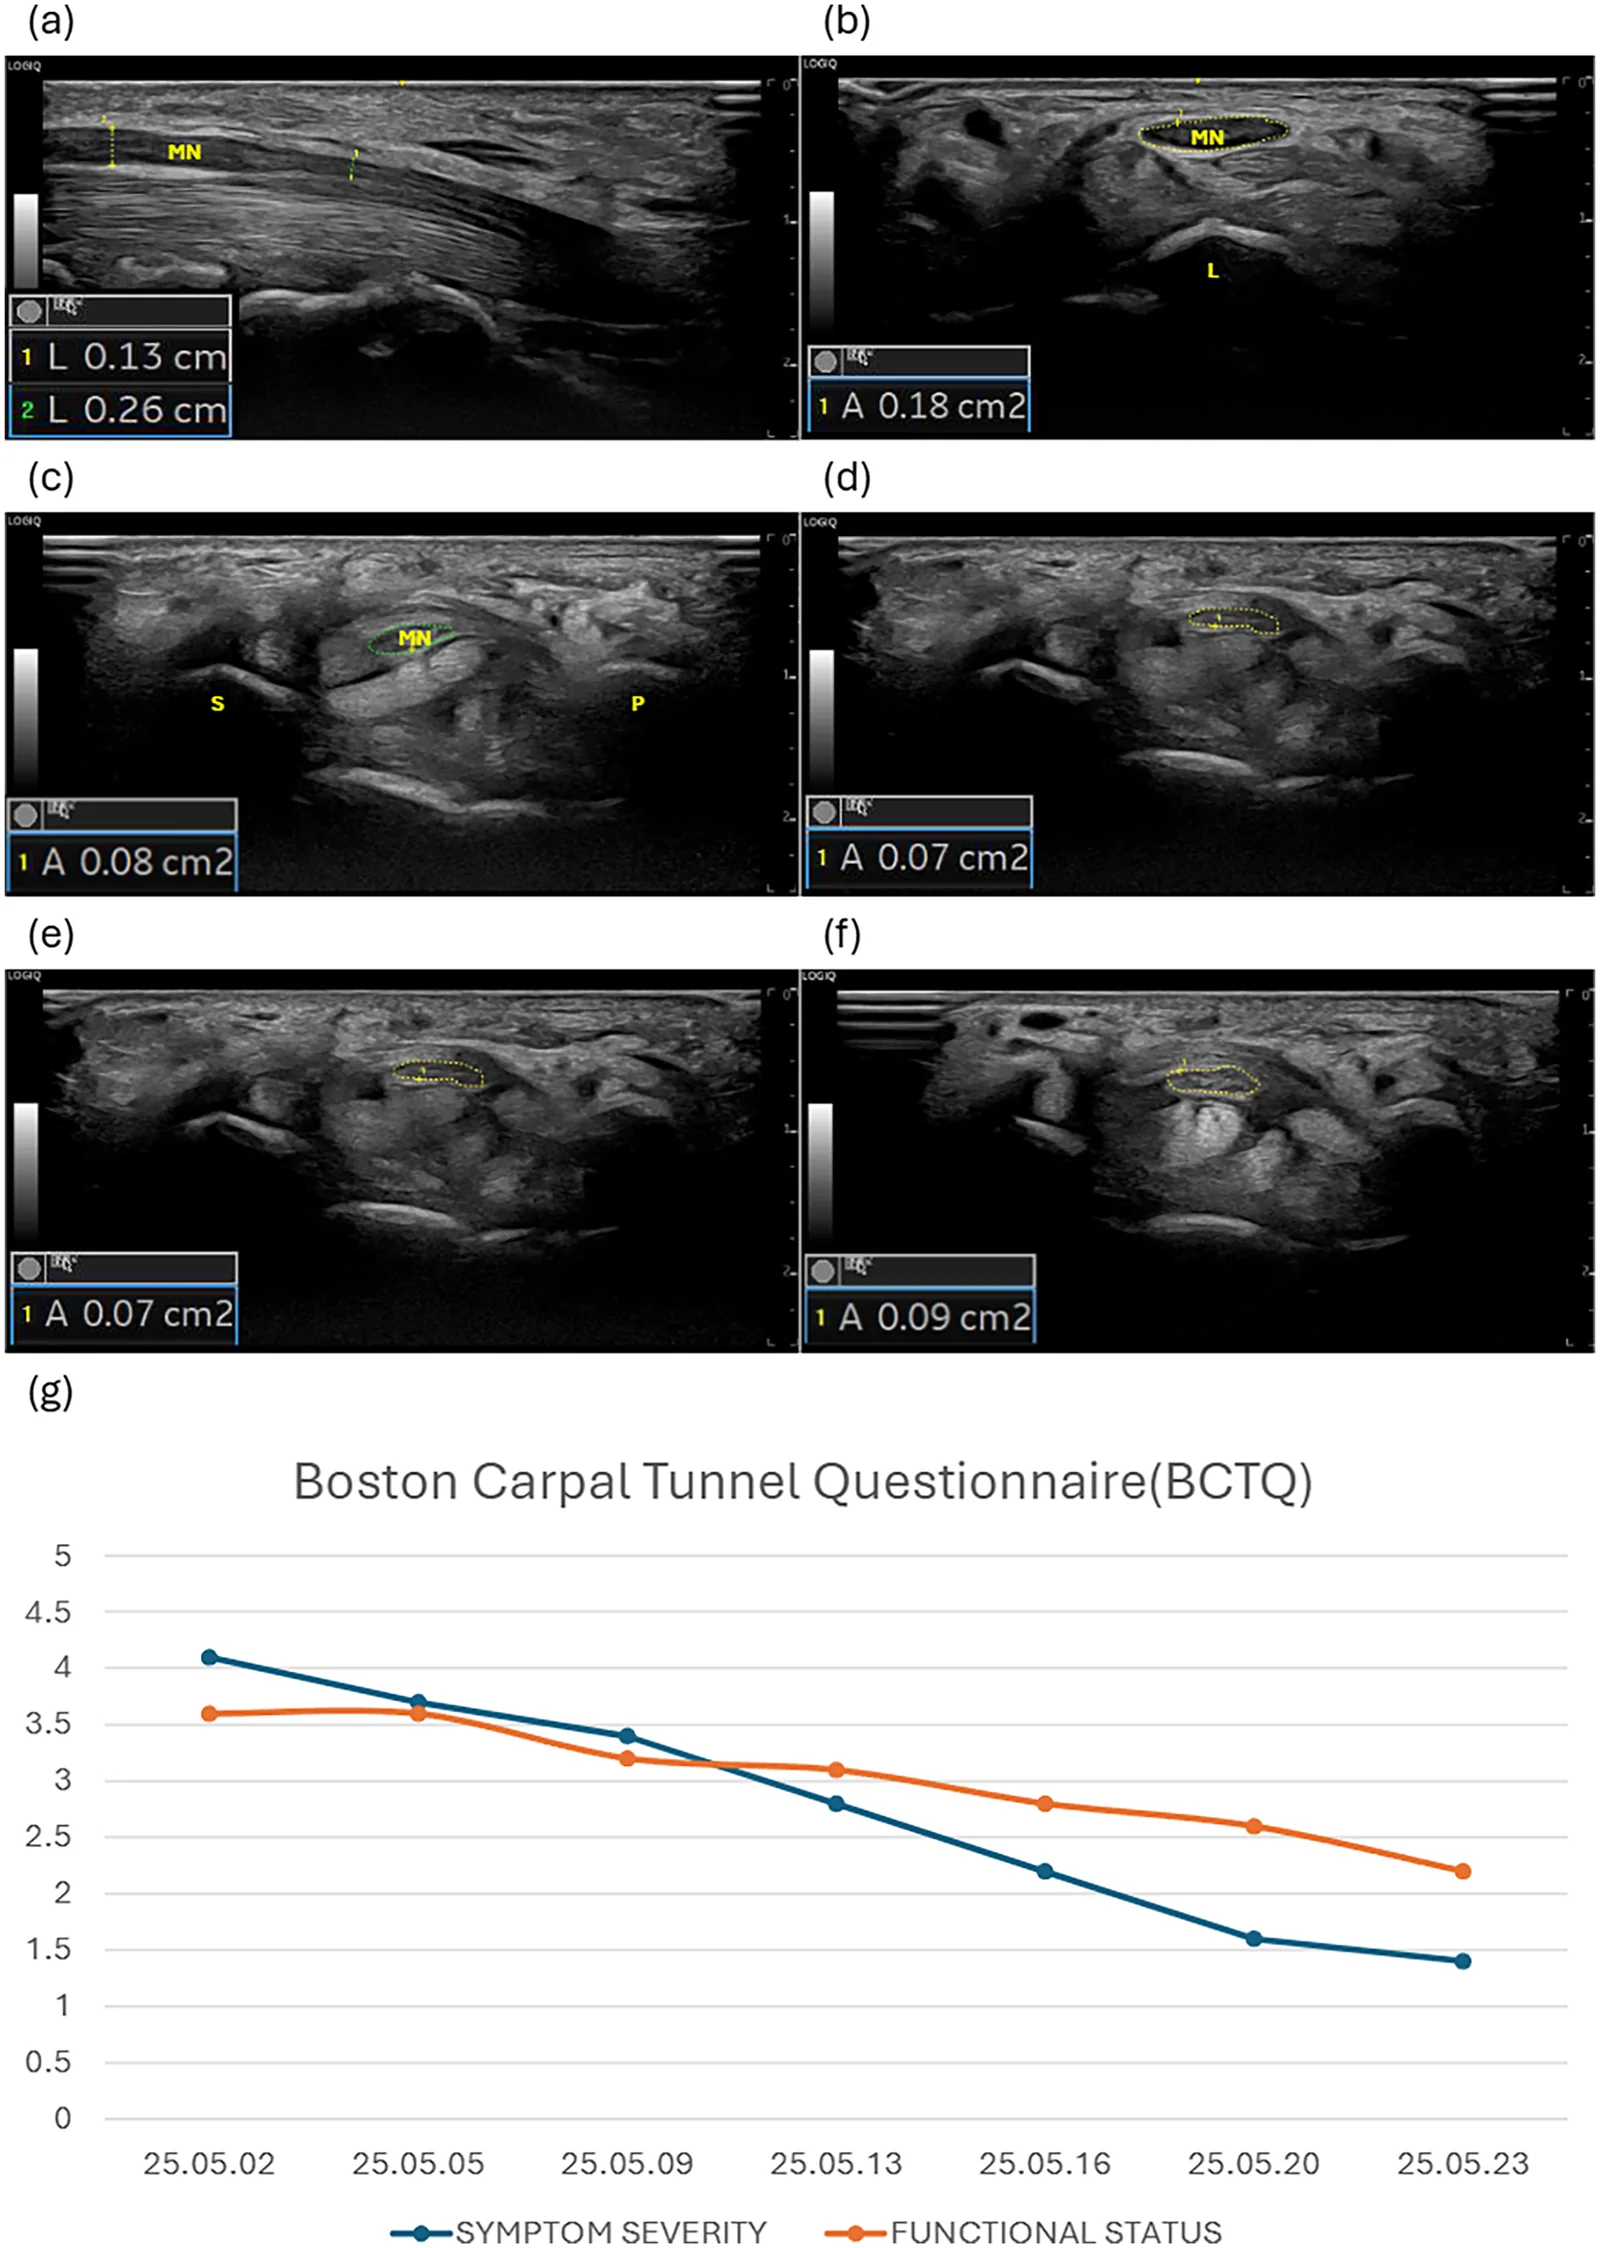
Ultrasound findings and clinical outcomes in case 2. (a) Longitudinal ultrasound image of the median nerve showing a notch sign. (b) Transverse ultrasound image taken at the lunate level demonstrating an increased cross-sectional area (CSA) of the median nerve (18 mm^2^). (c) Transverse ultrasound image at the proximal carpal tunnel with the fingers in a neutral position showing the location and CSA of the median nerve. (d) Transverse ultrasound image during finger flexion demonstrating ventral displacement and flattening of the median nerve with a reduced CSA (8 mm^2^ → 7 mm^2^). (e) Pre-treatment ultrasound image showing the CSA of the median nerve measured at the proximal carpal tunnel (7 mm^2^). (f) Post-treatment ultrasound image demonstrating an increased CSA (9 mm^2^) and improved morphology of the median nerve following ultrasound-guided hydrodissection. (g) Changes in Boston Carpal Tunnel Questionnaire (BCTQ) scores during the treatment period, showing progressive improvements in symptom severity and functional status. CSA, cross-sectional area; L, lunate; MN, median nerve; P, pisiform; S, scaphoid.
